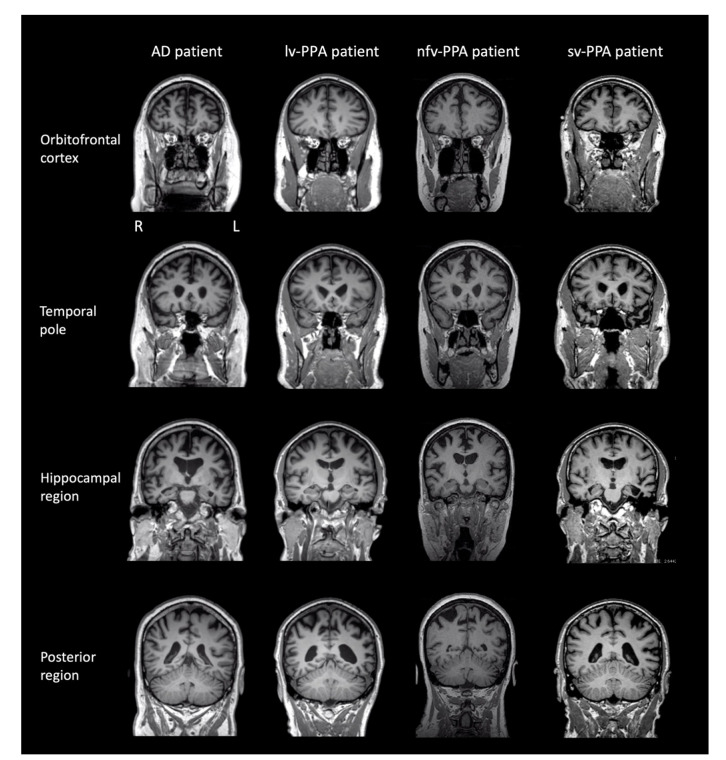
Brain T1 magnetic resonance images (MRI) of patients at the time of neuropsychological testing. Note: L = left; R = right. Brain images are presented in the coronal plane. lv-PPA patient: Mild generalised cortical atrophy was evident, slightly more prominent on the left than the right, extending posteriorly to involve the parietal lobes. There was marginally greater atrophy in the peri-insular region on the left than the right. nfv-PPA patient: Mild generalised cortical atrophy with particular involvement of the left peri-insular region anteriorly. sv-PPA patient: Severe atrophy of the anterior temporal pole bilaterally, but much worse on the left than the right. AD patient: Mild-moderate generalised cortical atrophy with involvement of the mesial temporal lobes.
